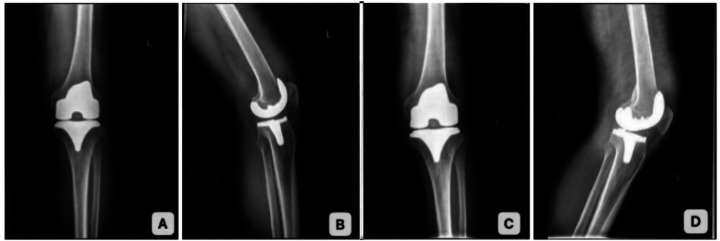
(A,B) Anteroposterior and lateral x-ray films of the left knee joint after total knee arthroplasty; (C,D) anteroposterior and lateral x-ray films of the left knee joint with infection after total knee arthroplasty.
